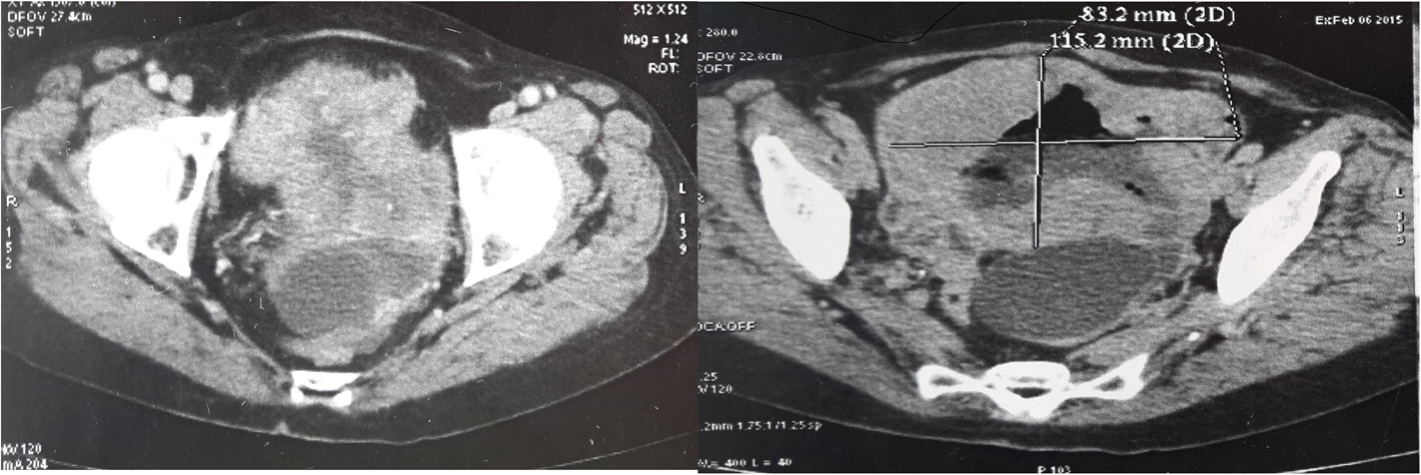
Computed tomography scan showing a pelvic mass measuring11 × 9 cm involving the distal small bowel loops, the bladder dome, and the uterine body without peritoneal effusion
